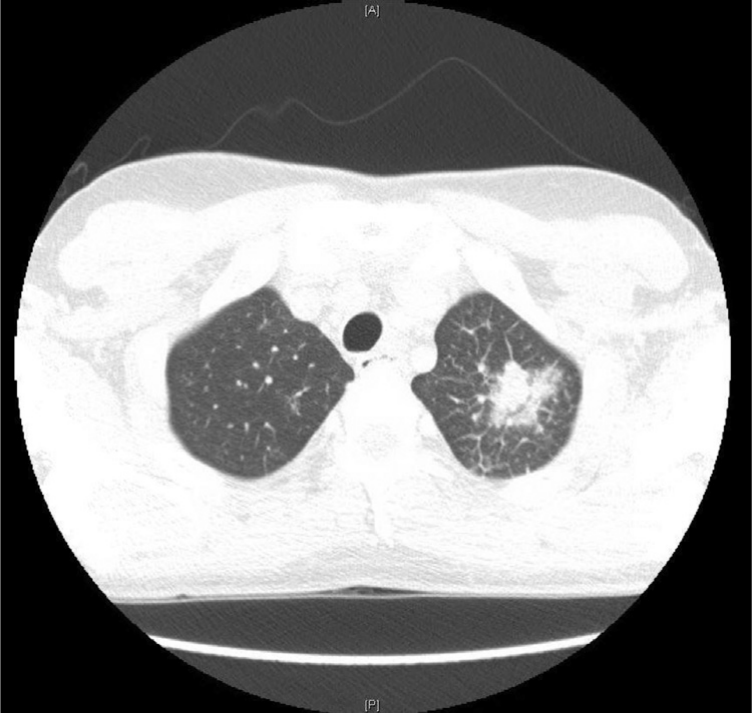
CT chest ordered for hypoxemia and showed a nodule in the left upper lobe.
